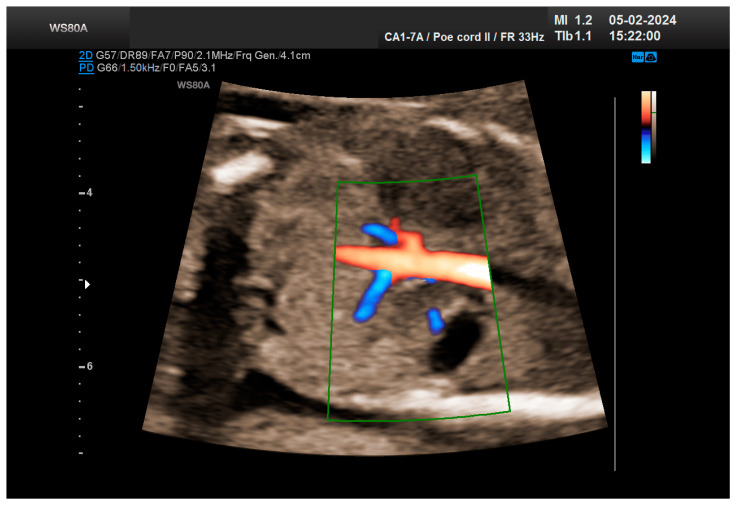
The vascularization of the “renal mass” seems to originate in the aorta. Green box—color Doppler window.
